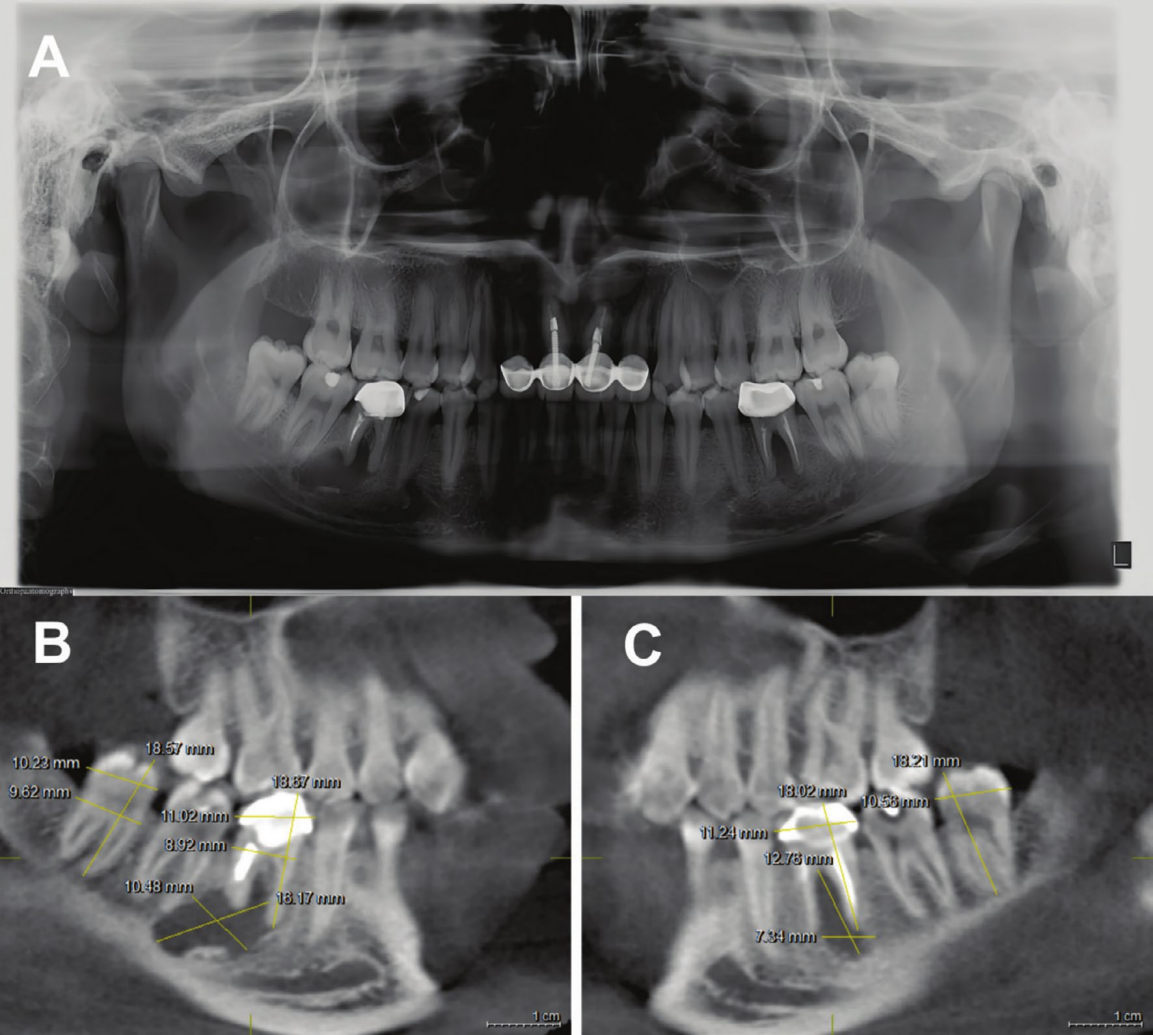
Preoperative radiographic conditions of recipient sites (#36, 46) and donor teeth (#38, 48). (A) Curved panoramic view. (B, C) Sagittal plane view.
